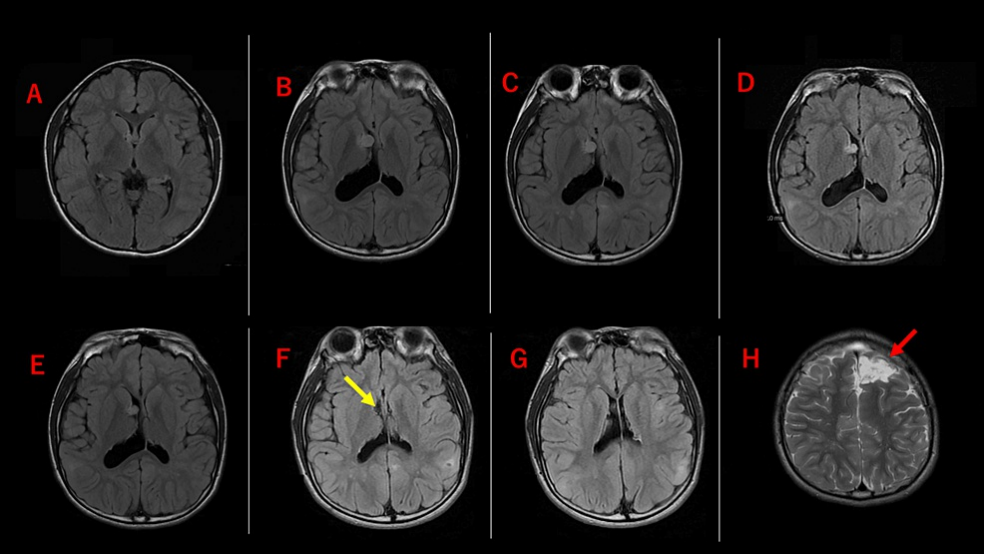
Changes in the size of the SEGA and ventricles over time Figures A, B, C, D, and E show preoperative head MRI, and Figures F, G, and H show postoperative head MRI. In June X-1, SEGA present near the foramen of Monro in the right lateral ventricle was small and no ventricular enlargement was identifiable (A). A follow-up MRI approximately one year later in May X showed that both the SEGA and bilateral ventricles had enlarged with a right-side predominance (B). There was a discussion about whether to remove the SEGA surgically or to manage it with mTOR inhibitors; however, since there was no increased intracranial pressure, we used mTOR inhibitors. No symptoms of increased intracranial pressure were noted at that time, and hence mTOR inhibitor was first used. After approximately five months of treatment with mTOR inhibitor, in October X, the SEGA had reduced in size, but the ventricle size remained basically unchanged (E). Also shown are MRIs that were taken one month (C) and three months (D) after starting the mTOR inhibitor. A transcallosal approach was therefore used to resect the SEGA. Resection of the left frontal lobe, identified as a possible epileptogenic zone in a subdural electrode study, was performed simultaneously (H). The SEGA was completely resected (F), and ventricle size normalized (G). The disappearance of epileptic seizures suggests that the epileptogenic zone was successfully resected MRI: magnetic resonance imaging; mTOR: mammalian target of rapamycin; SEGA: subependymal giant cell astrocytoma
